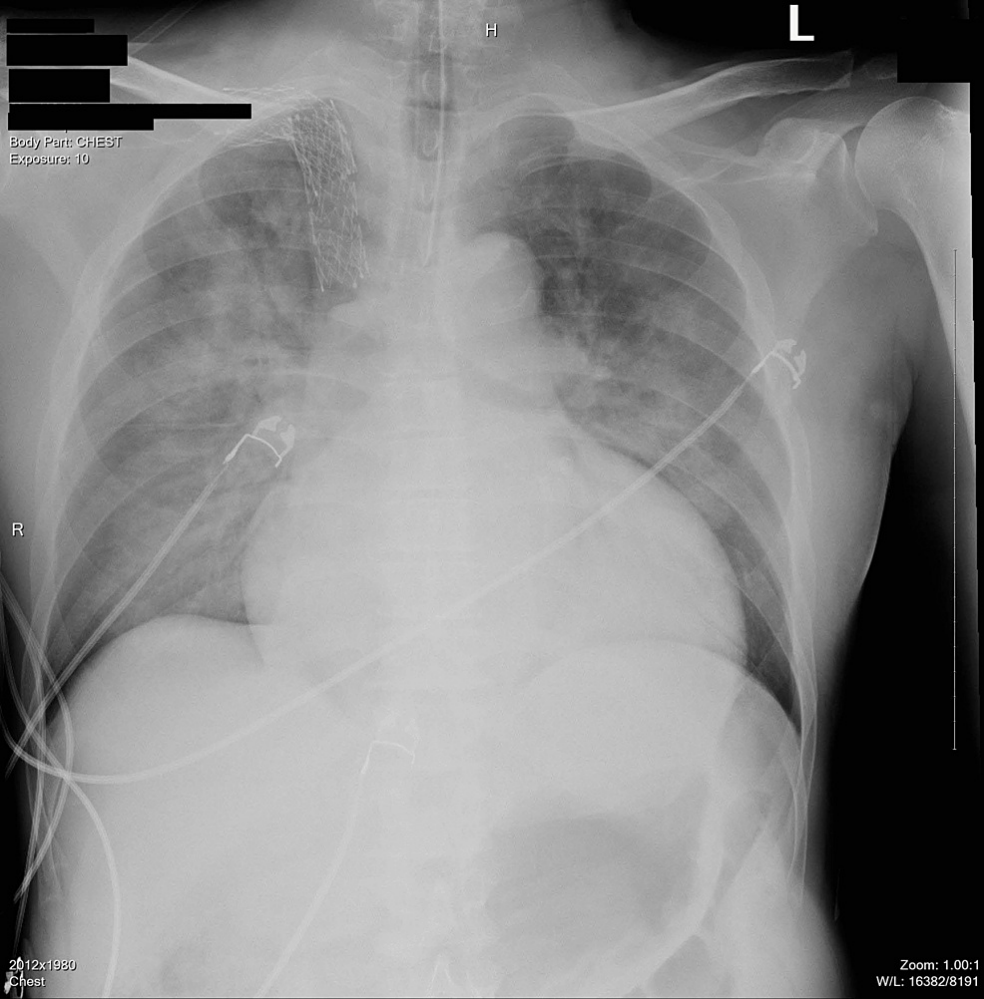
Chest X-ray showing bilateral interstitial, fluffy infiltrates, and ground-glass opacities consistent with pulmonary edema and cardiomegaly.
